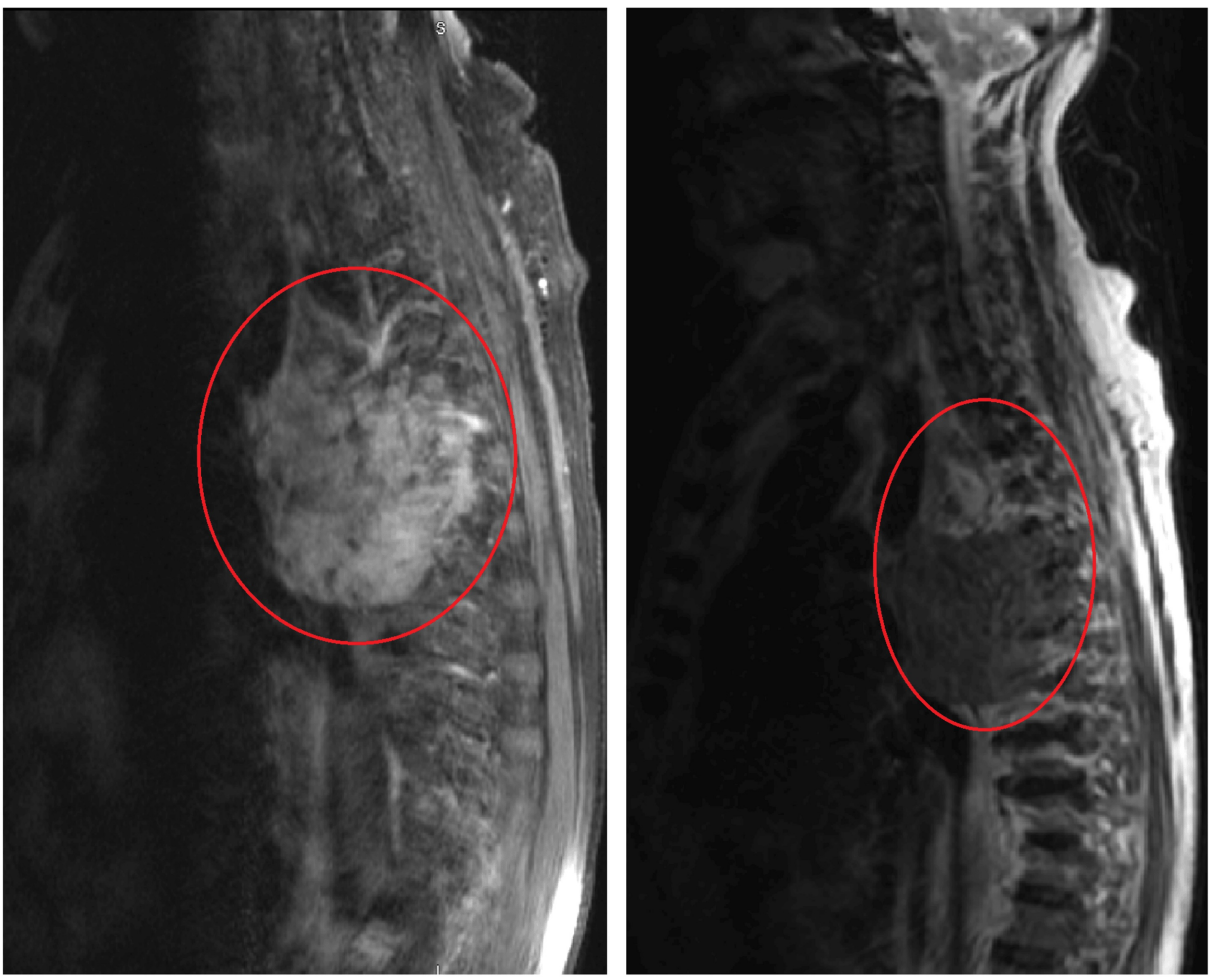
MRI of the thoracic spine with and without contrast The large mass indicated by the red circle is located in the right hemithorax encroaching on the right neural foramina at T5-T6 and T6-T7, contributing to severe neural foraminal stenosis at T5-T6 on the right. No significant spinal canal stenosis. MRI: magnetic resonance imaging
